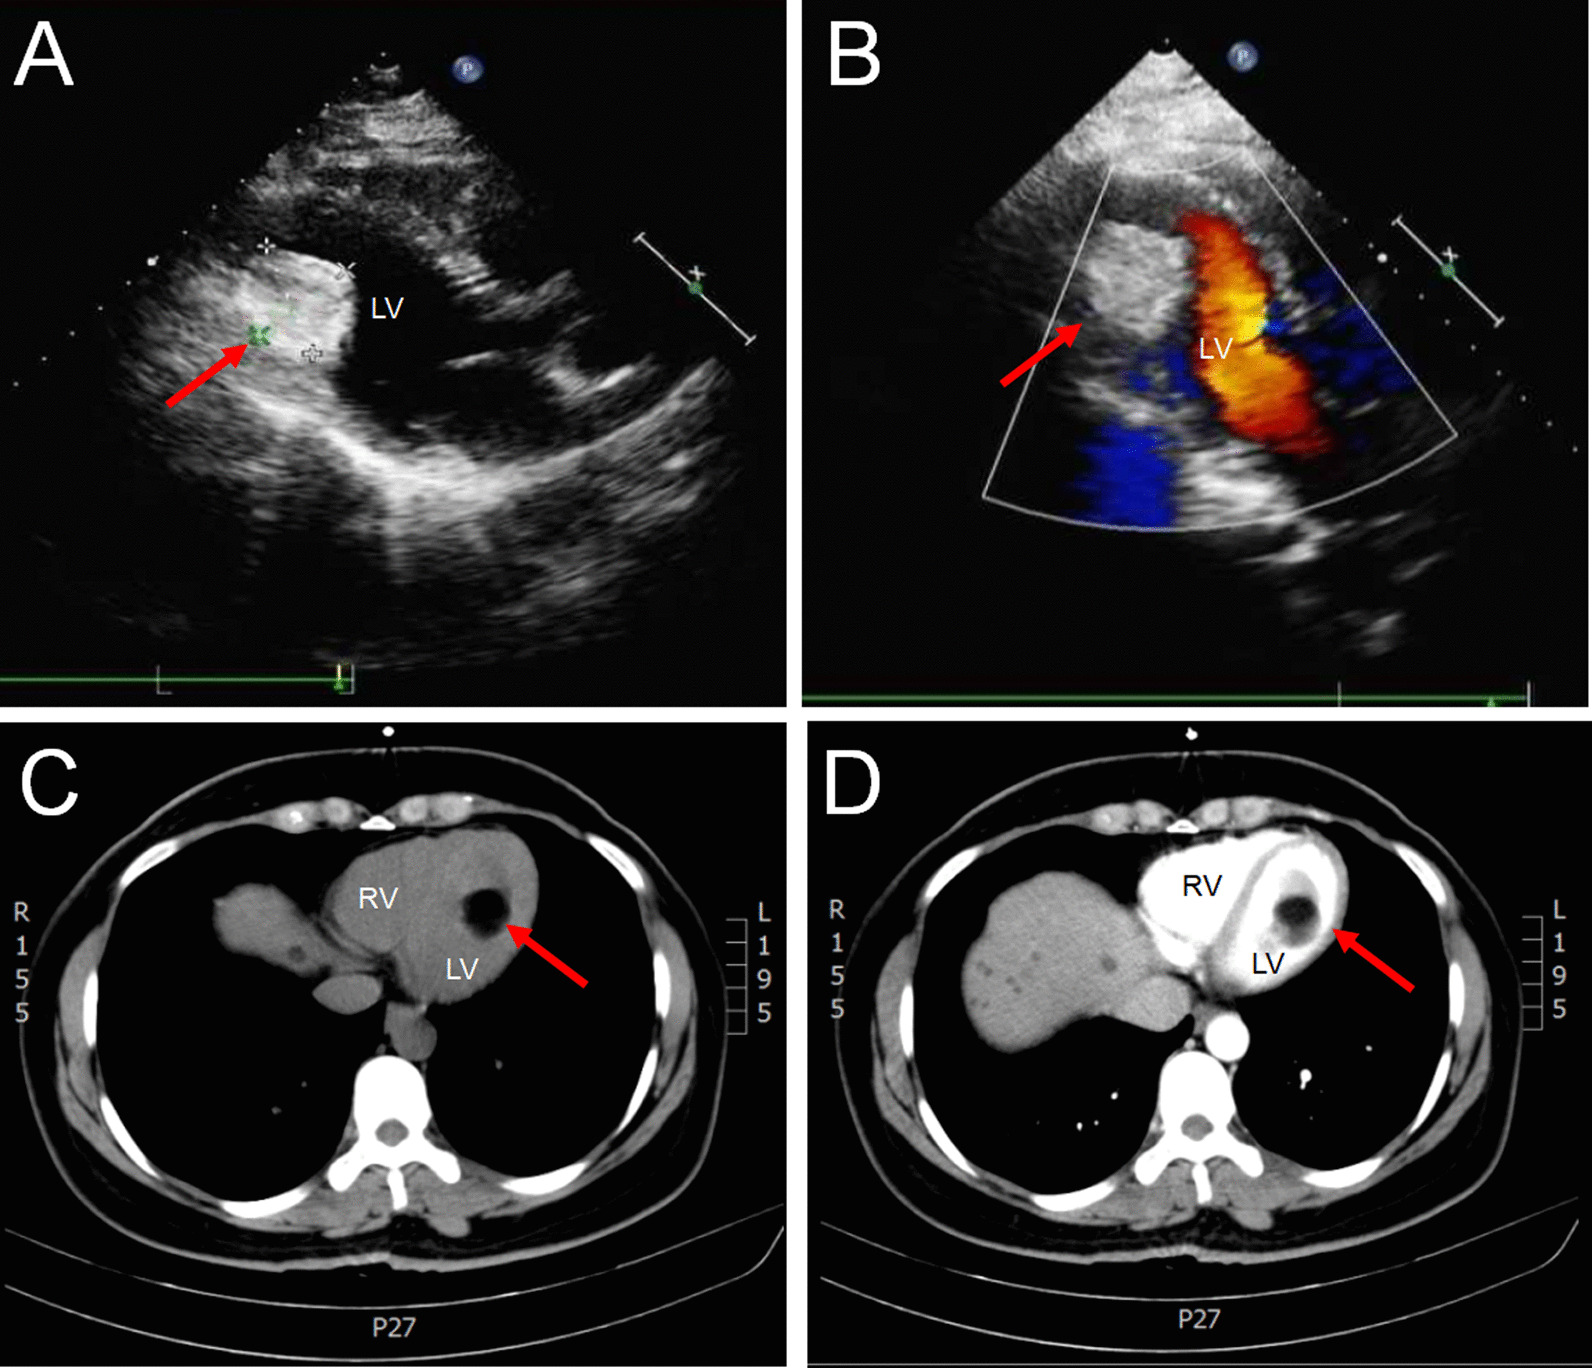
Echocardiography revealed an echo-bright mass in the LV (A). Color-flow Doppler echocardiography showed no significant effect on the peripheral valves and hemodynamics (B). Cardiac computed tomography was performed and revealed a quasi-circular low-density focus with a clear boundary and regular shape in the LV (C and D). LV: left ventricle; RV: right ventricle
